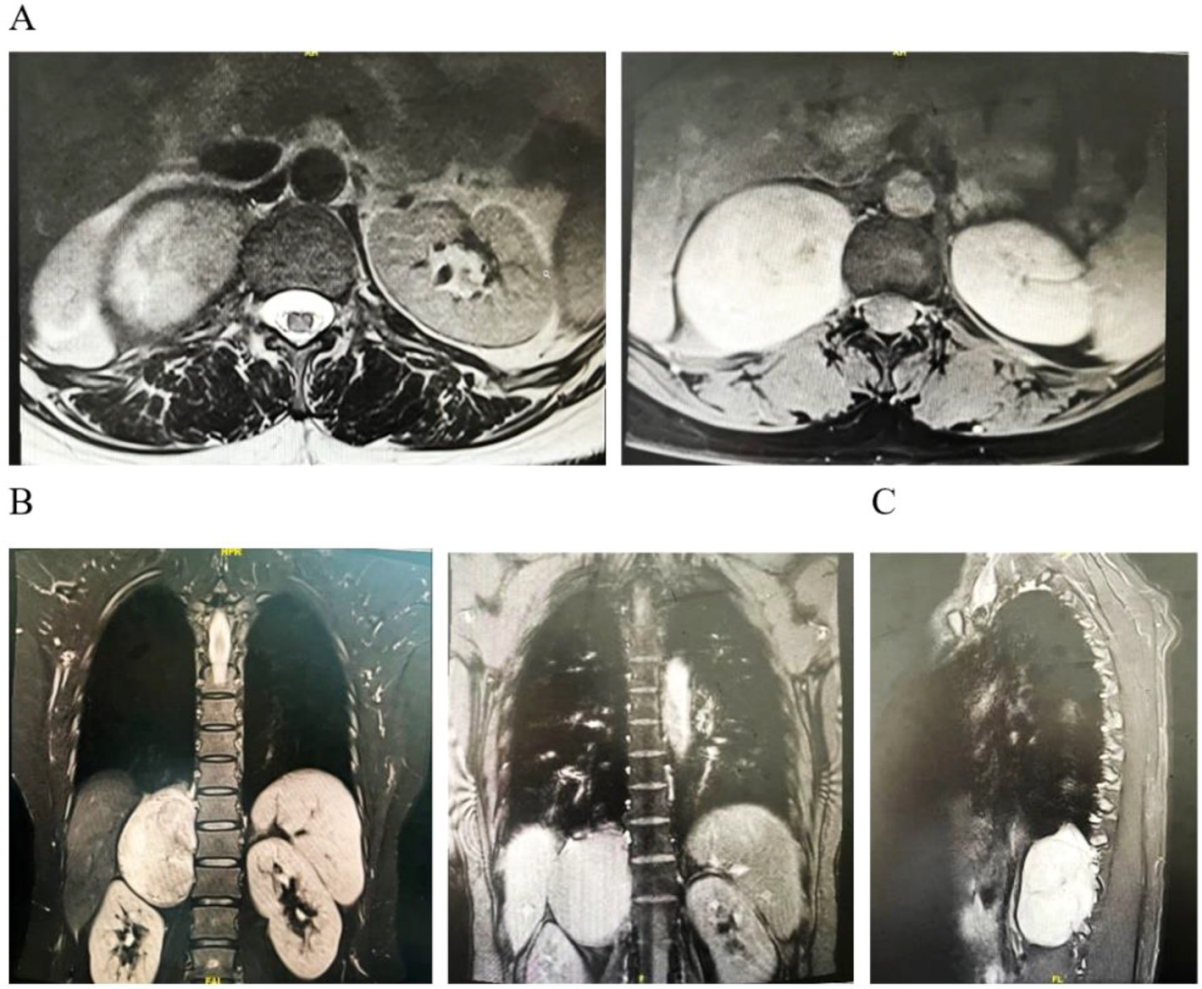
Representative images of MRI scans. (A) Cross-sectional scan. (B) Coronal scan. (C) Sagittal scan.
